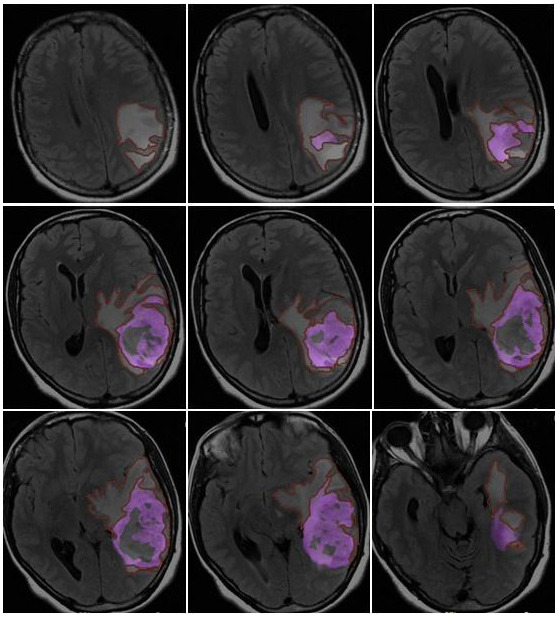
Edema/infiltration preoperative volume (T2/FLAIR-PV), evaluated manually on axial hybrid sequences resulting from FLAIR (slice thickness: 5 mm; slice spacing: 5.5 mm) and CE-T1w in order to exclusively measure the edema/infiltration component, excluding the tumor (enhancing mass) previously assessed with CE-PTV and CE-RTV measurements.
